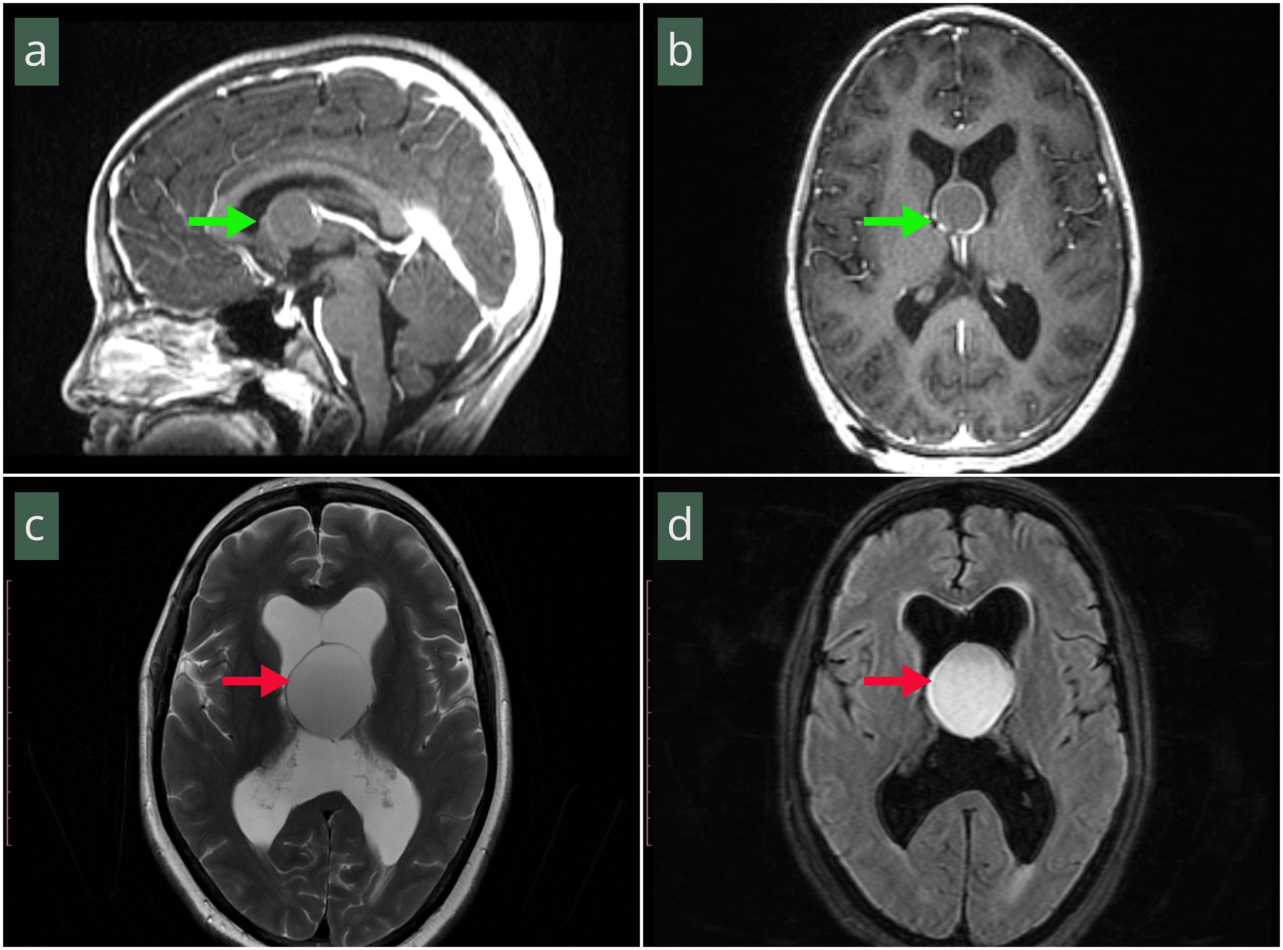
(a, b) Sagittal and axial post-contrast T1-weighted MRI demonstrating thin rim enhancement of the cyst capsule with isointense intra-cystic fluid. (c) Axial T2-weighted MRI showing an iso- to hyperintense, round encapsulated lesion obliterating the third ventricle (red arrow). (d) Axial T2-weighted MRI showing a hyperintense, round lesion obliterating the third ventricle (red arrow). MRI: magnetic resonance imaging
